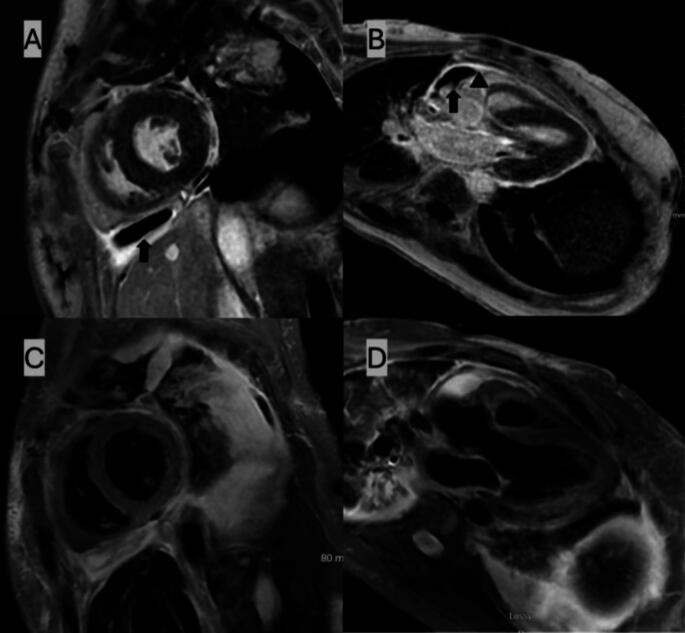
A diagnosis of effusive constrictive pericarditis was made via CMR. (A) short axis image showing thickened pericardium measuring 3.2 mm with gadolinium enhancement (arrowhead) and pericardial effusion (arrow); (B) long axis image showing thickened pericardium (arrowhead) with loculated pericardial effusion (arrow) and aortic regurgitation; (C, D) T2-weighted dark blood images showing hyperintense pericardial effusion without myocardial edema
